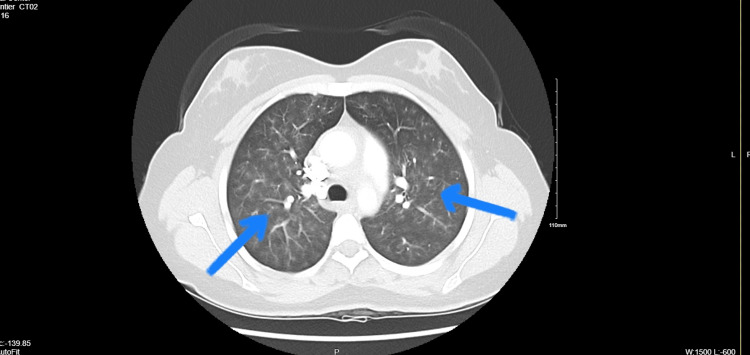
CT chest showing diffuse centrilobular bilateral ground-glass (blue arrows) opacities suggestive of inflammatory vasculitis.
